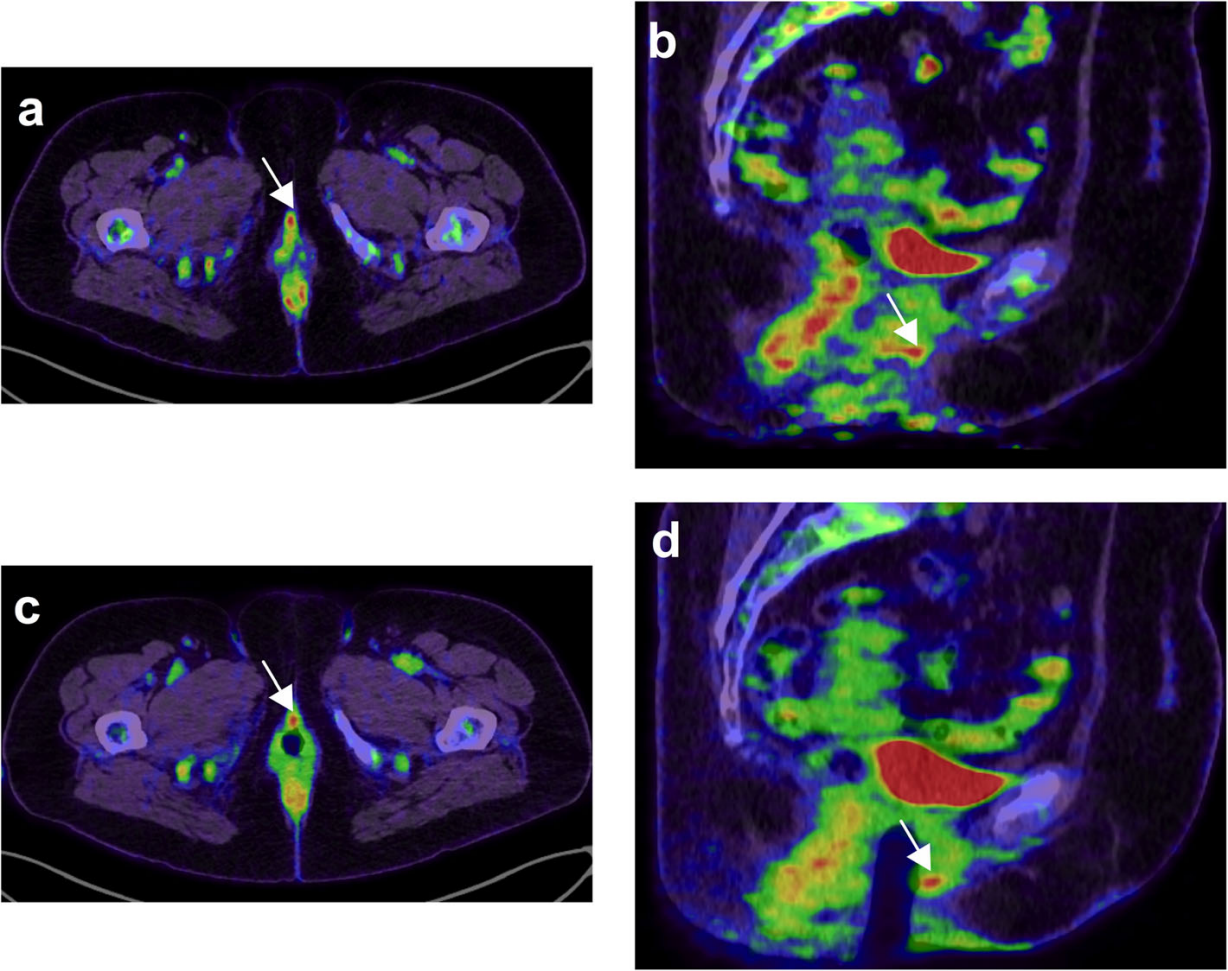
61-year-old woman with suspicion of recurrence for a vulvar melanoma. From left to right, PET/CT fused axial (a and c) and coronal (b and d) slices. Patient was scanned before and after introduction of a vaginal tampon because of an indeterminate pelvic focus (white arrow). The images after introduction of the vaginal tampon allowed precise localization of the FDG focus on the anterior vaginal wall
